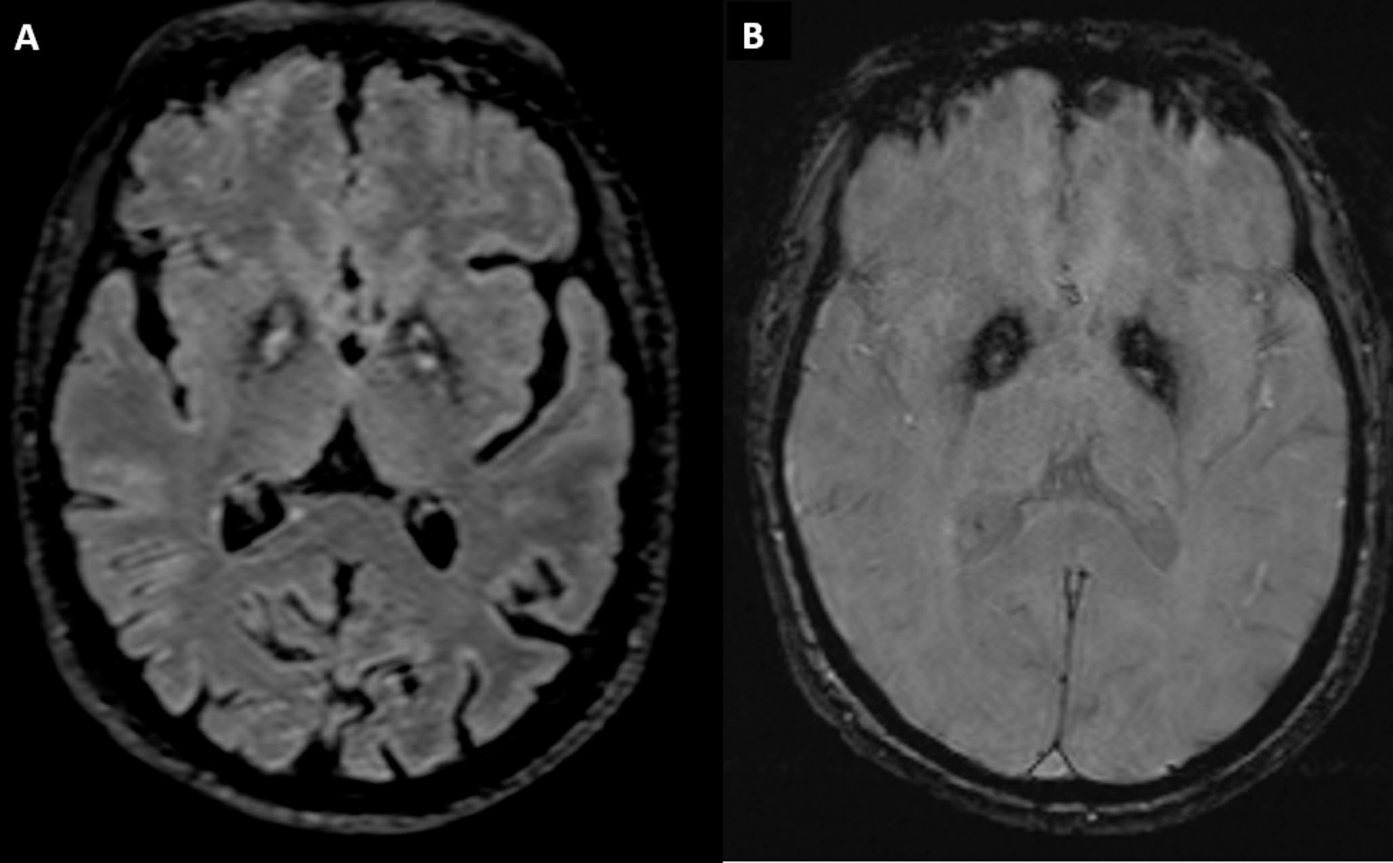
Brain MRI Imaging. (A) Axial-slice of T2-FLAIR weighted sequences with the classical “Eye of the tiger sign” defined by a bilateral central region with hyperintense signal surrounded by hypointensity in the globus pallidus. (B) Axial slice of Susceptibility Weighted Imaging sequences showing susceptibility artifact (low signal) in corresponding areas from iron deposition
